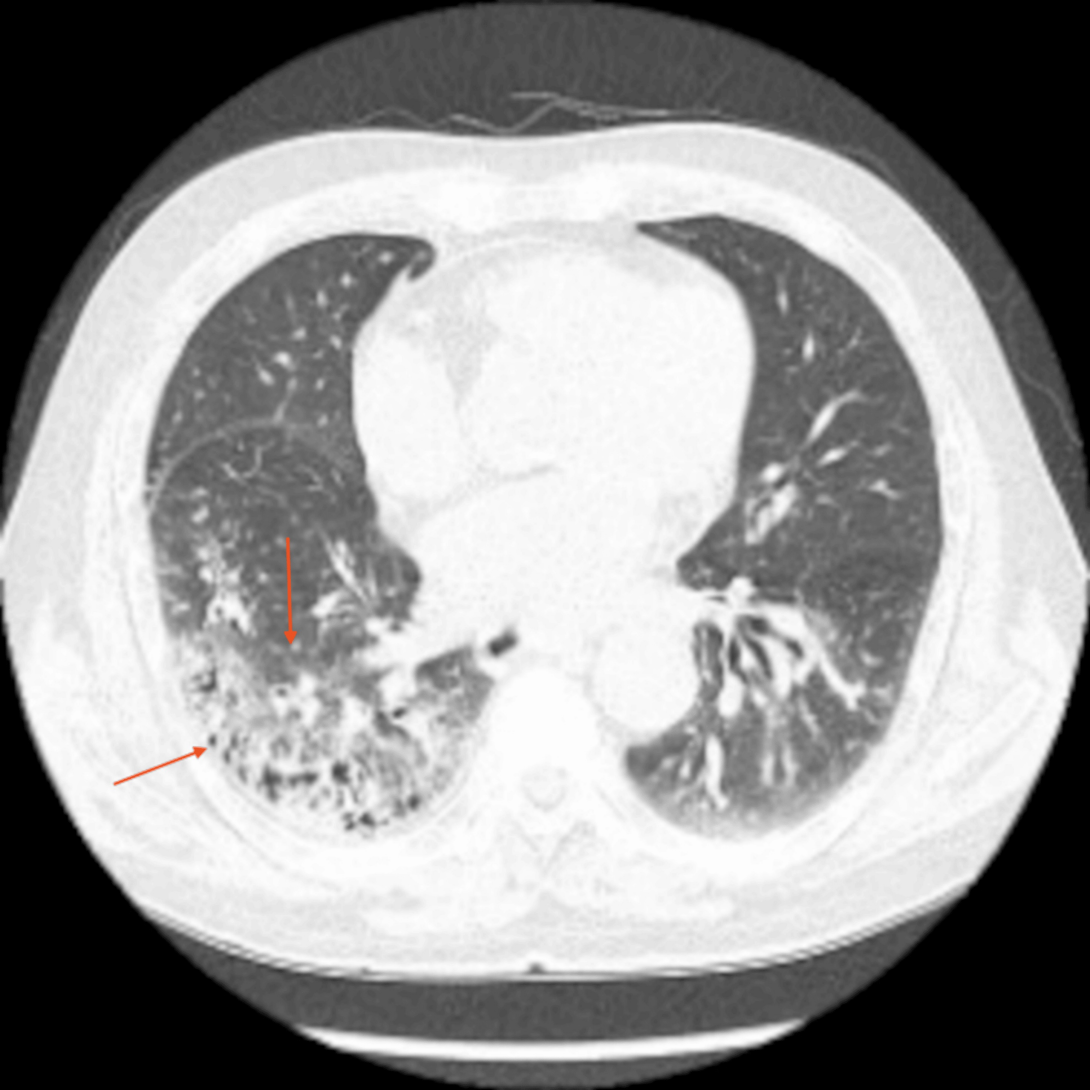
Chest CT suggesting aspiration pneumonia. The red arrow highlights consolidation and ground-glass opacity in the right lower lobe. Axial view of chest CT demonstrating an area of consolidation and ground-glass opacity in the right lower lobe, consistent with aspiration pneumonia. The red arrow marks the region of interest.
